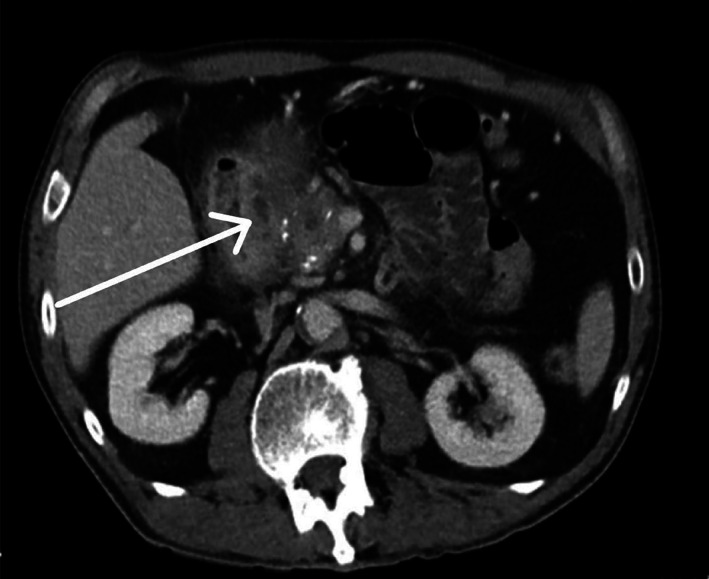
Axial CT image of groove pancreatitis with paraduodenal cysts and chronic calcific changes in the pancreatic head.
